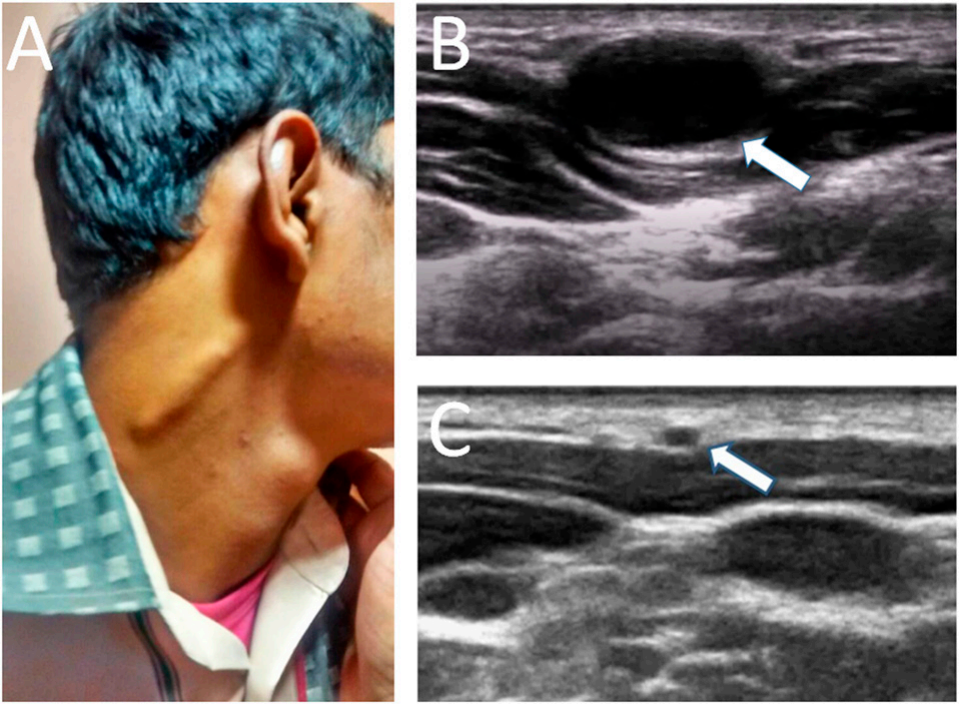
Clinical and ultrasound findings in greater auricular neuropathy (GAN) in Hansen’s disease. (A) Cord-like enlargement of the right GAN. Thickening of the right GAN was confirmed on B-mode high-resolution ultrasonography (B). Color Doppler ulrasononography revealed increased perineural vascularity. For reference, the normal ultrasonographic appearance of the left GAN is shown in panel C. This figure appears in color at www.ajtmh.org.
